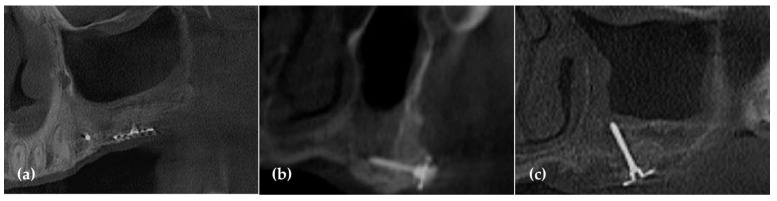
(a) The panoramic image of the CBCT after 5 months of healing shows successful vertical ridge augmentation and maxillary sinus augmentation; (b) Remarkable horizontal ridge augmentation is observed in the premolar region; (c) The cross-sectional CBCT image demonstrates both horizontal and vertical ridge augmentation.
